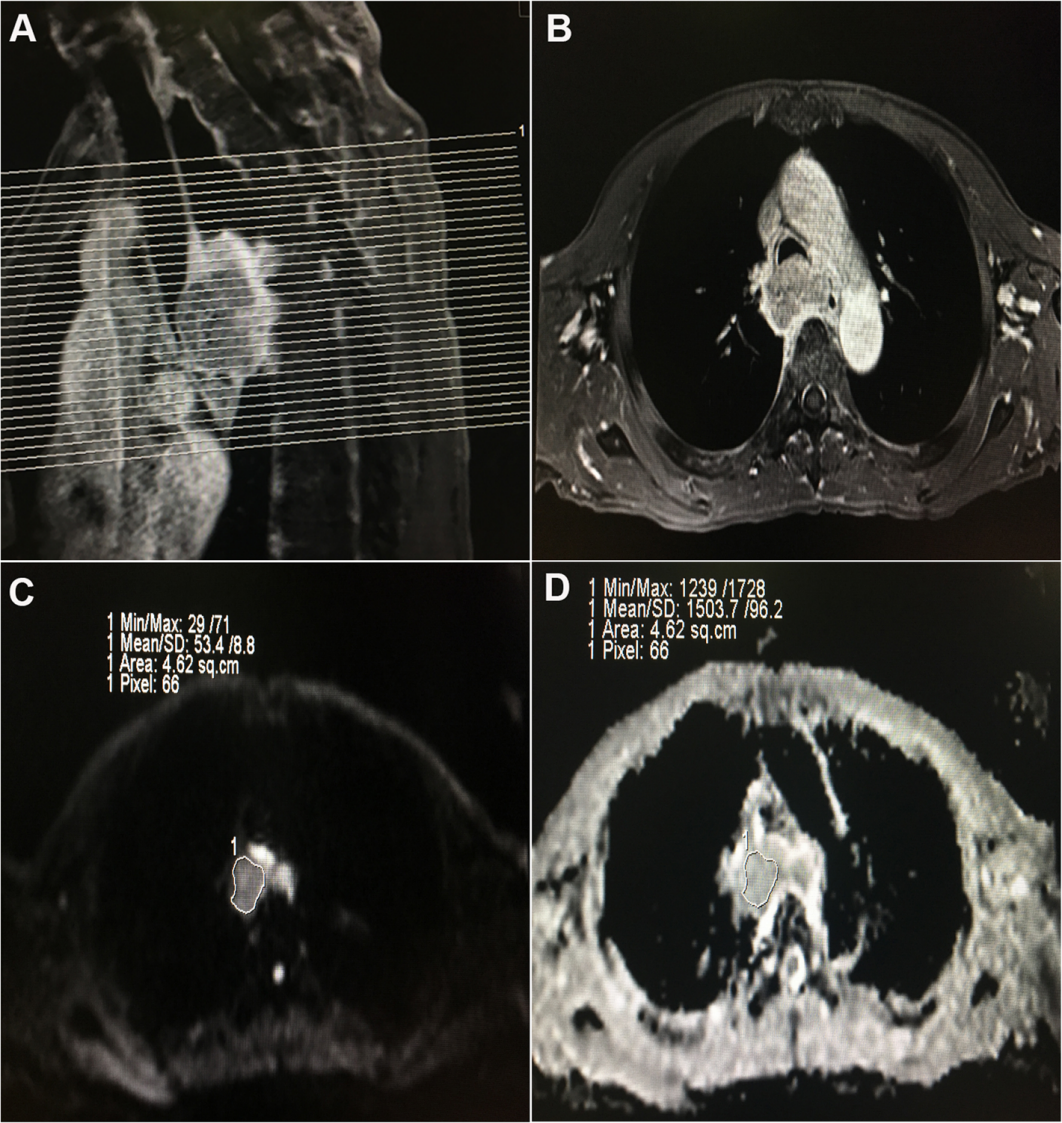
Example of ADC measurements of a primary tumour in a patient diagnosed with esophageal carcinoma before treatment. a. A sagittal view of T1 with contrast-enhanced (T1 + C) image with position line for the primary tumour. b A transverse view of the T1 + C image from the one of three continuous sections with maximal diameter based on the transverse and sagittal view. c. A region of interest (ROI) was contoured manually in the selected section of the DWI image, which was obtained at a b-value of 1000 s/mm^2^, and the ROI was then copied and pasted onto the ADC map (d), and the ADC value of the selected section was automatically calculated
